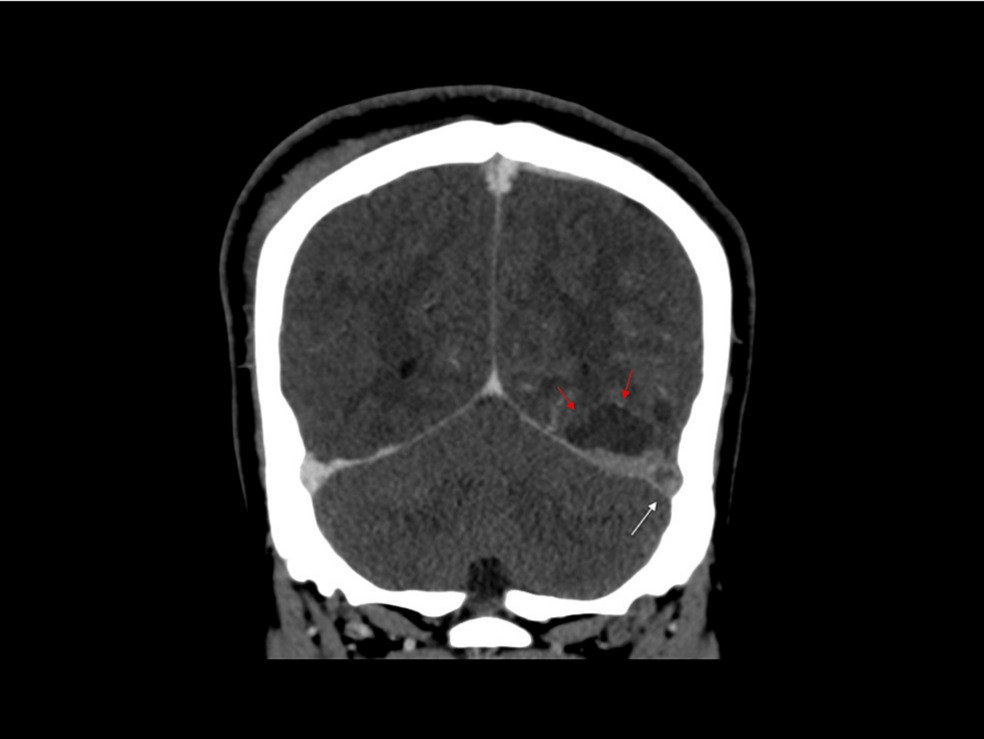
Contrast-enhanced computed tomography venogram White arrow: shows a filling defect at the left transverse sinus denoting thrombus; red arrows: associated left occipital lobe hypodensity in keeping with the infarction
